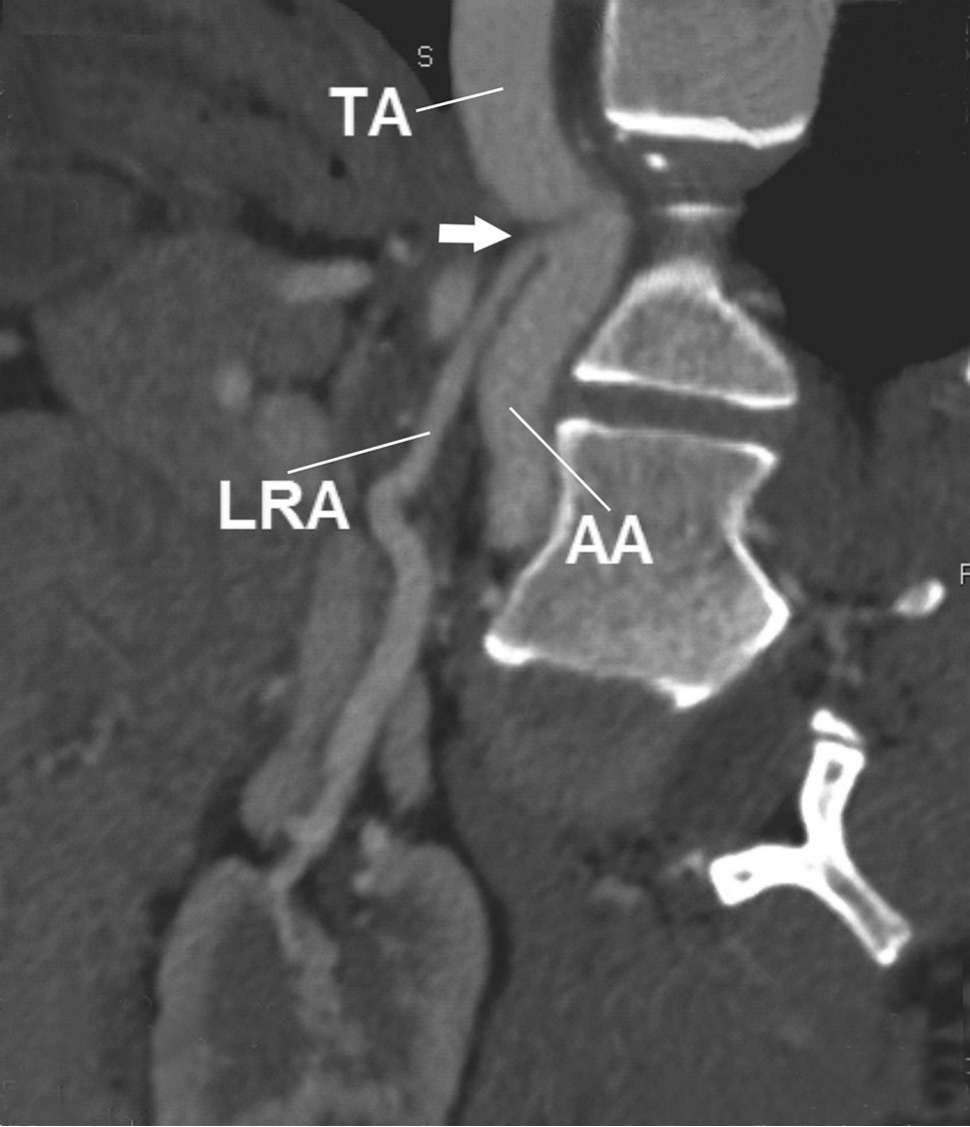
Abdominal computed tomography angiography, planar reconstruction. *Arrow* origin of left renal artery. *AA* abdominal aorta, *LRA* left renal artery, *TA* thoracic aorta
